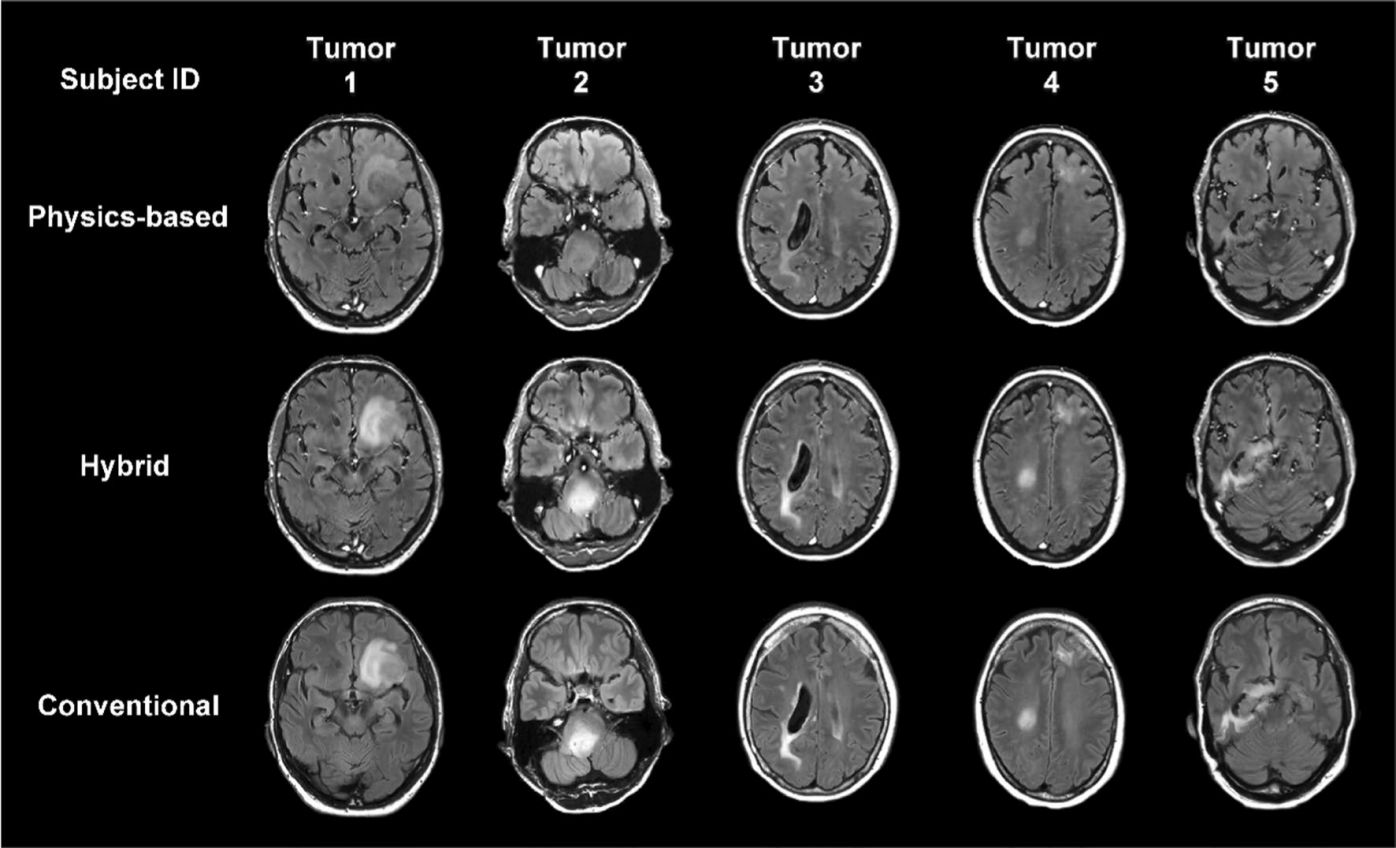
Comparison of FLAIR images synthesized according to the physics-based model, the hybrid method (12.5% of conventional FLAIR acquisition is used) and conventional FLAIR images. The axial slices of interest from the five tumor patients are shown
